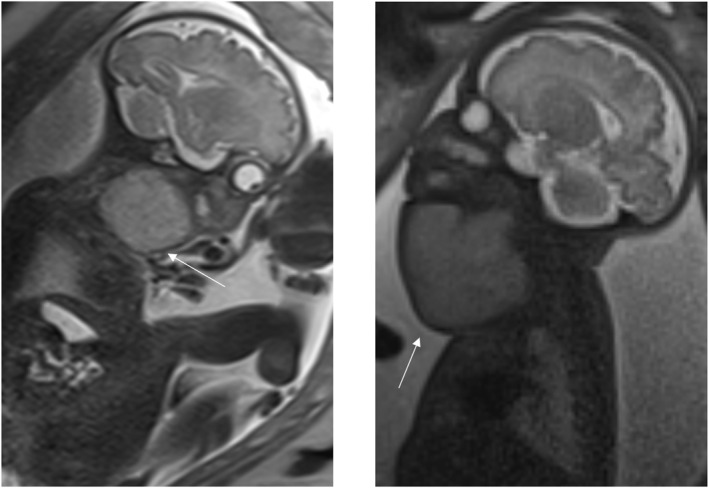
Fetal MRI images of rhabdomyosarcoma. T2 haste sagittal images of a 33 weeks fetus (left) and a 30 weeks fetus (right) both with a solid anterior neck mass causing airway compression/displacement.
